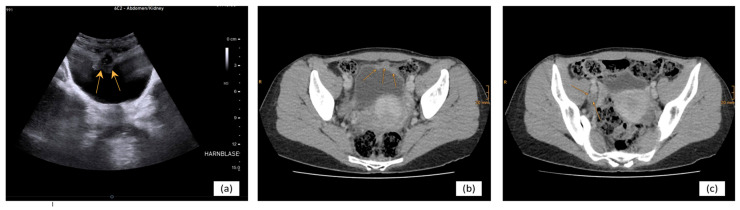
Preoperative cross-sectional imaging: Abdominal ultrasound performed prior to transurethral resection of the bladder demonstrates a well-defined mass at the bladder dome ((a), marked with arrows). Representative contrast-enhanced computed tomography images show a tumor arising from the urachal tract with extension into the bladder dome ((b), marked with arrows). A markedly enlarged lymph node is visible in the right pelvic region between the external iliac and obturator nodal stations, consistent with radiologically suspected nodal metastasis ((c), marked with arrows). No evidence of distant metastatic disease was identified on staging imaging.
